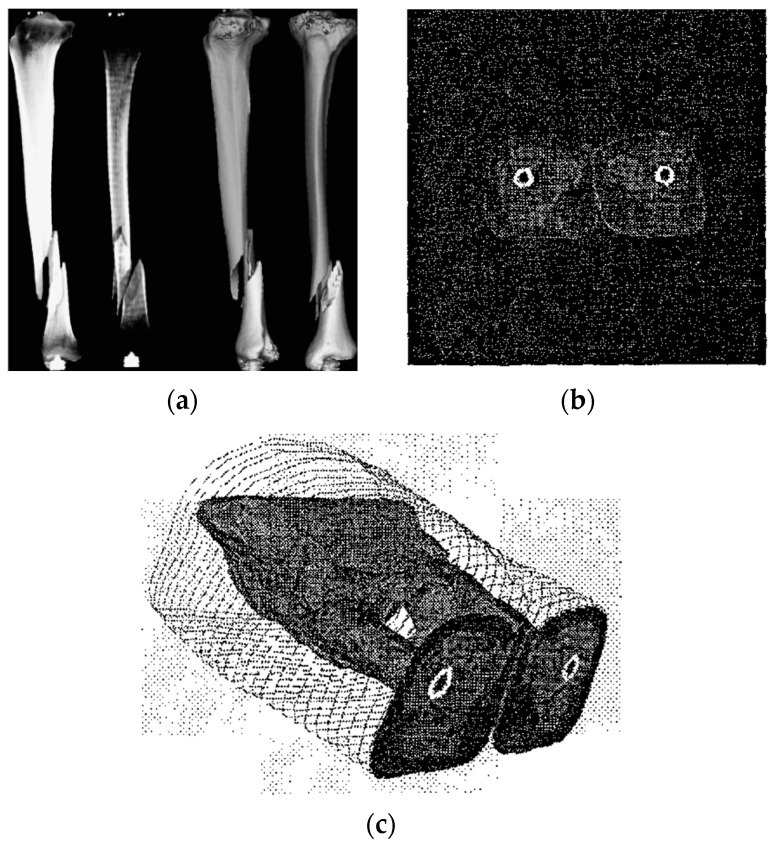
(a) Bone fracture displays in 3D visualization software, the Sobel-filter is used for detecting the outline of bone [11]; (b) CT image slides and (c) 3D visualization [4].
